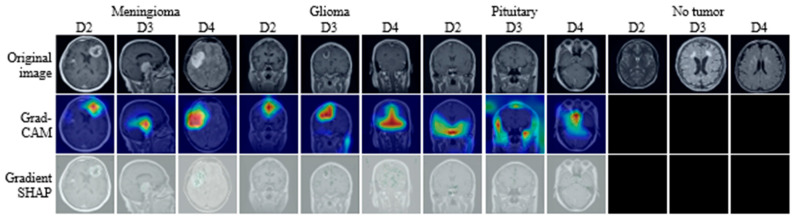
Grad-CAM++ and Gradient SHAP visualizations of ConvNeXt Base predictions with corresponding original MRI slices across tumor classes and datasets.
